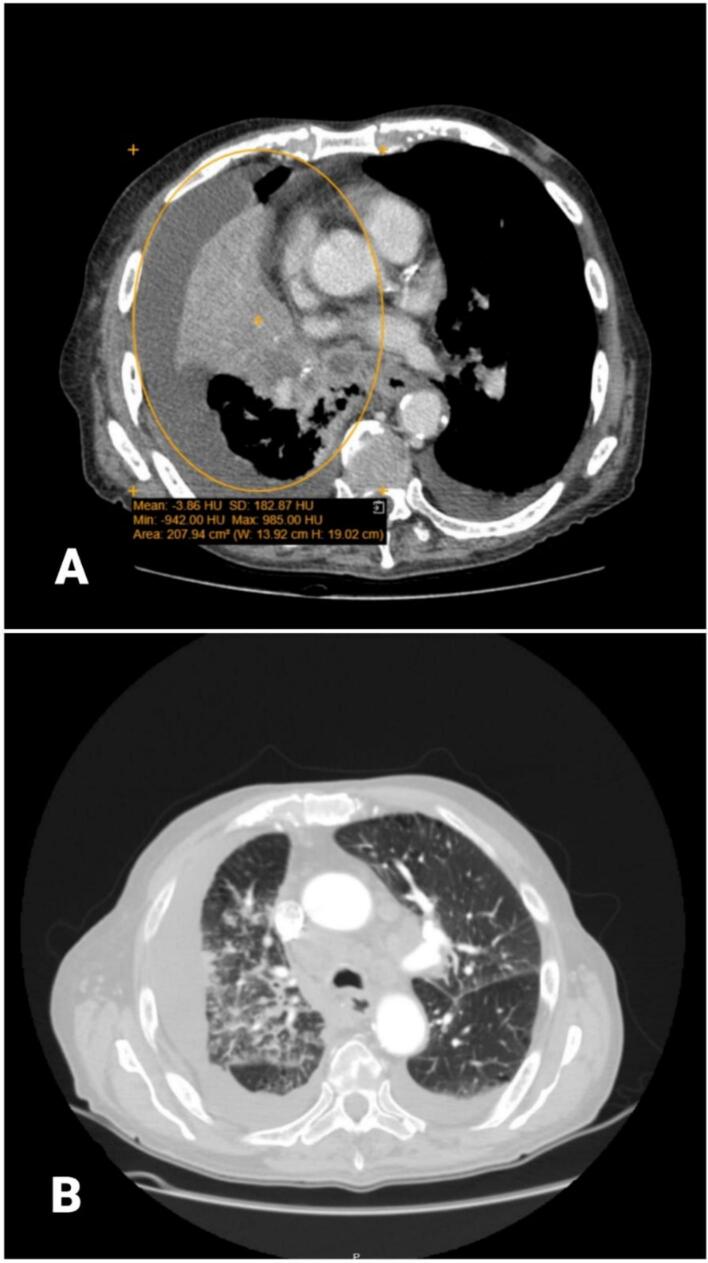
Axial CT scan with IV contrast showed A. Collapsed lung parenchyma in the right middle lobe and most of the right lower lobe. B. lymphangitis carcinomatosis.
